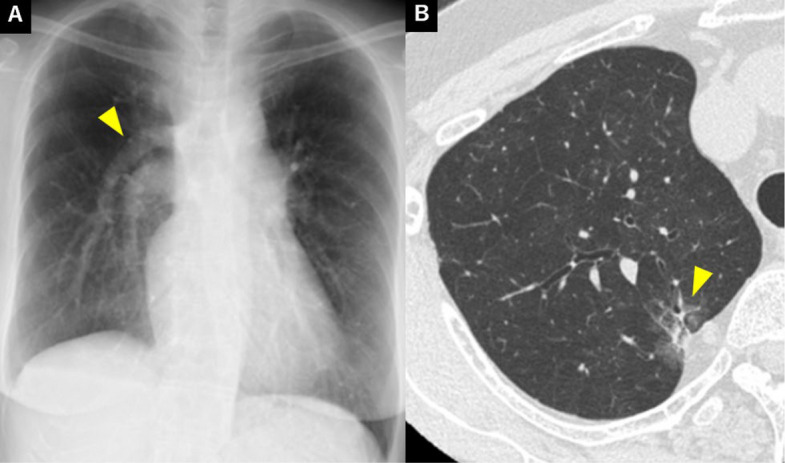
A Chest X-ray. An abnomal pulmonary venous shadow in the rigt lung field. B Chest CT. A 25 mm part solid nodule was detected in the RUL
